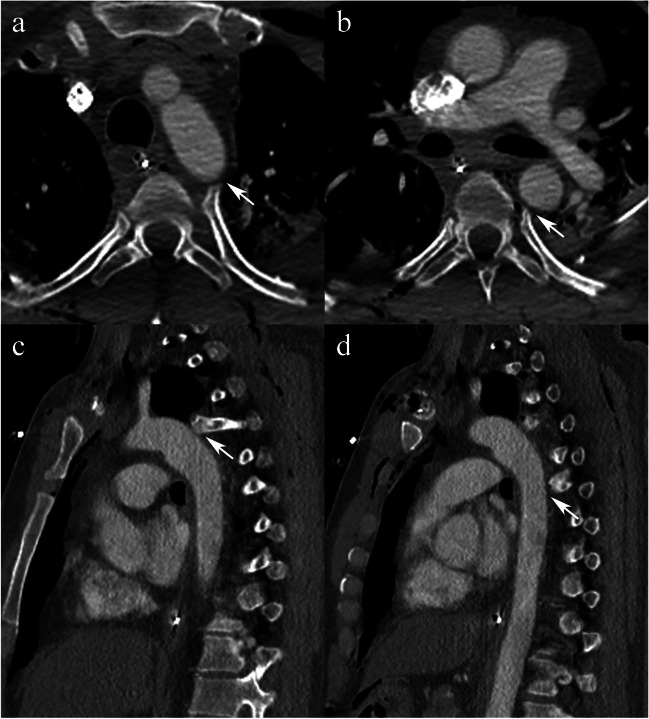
Initial post-trauma CT in 36-year-old male who was run over by a car, with axial (a) and sagittal (c) images demonstrating a left fourth costovertebral joint dislocation, and axial (b) and sagittal (d) images showing a left sixth costovertebral joint subluxation, with the heads of the ribs abutting the aorta (arrows)
